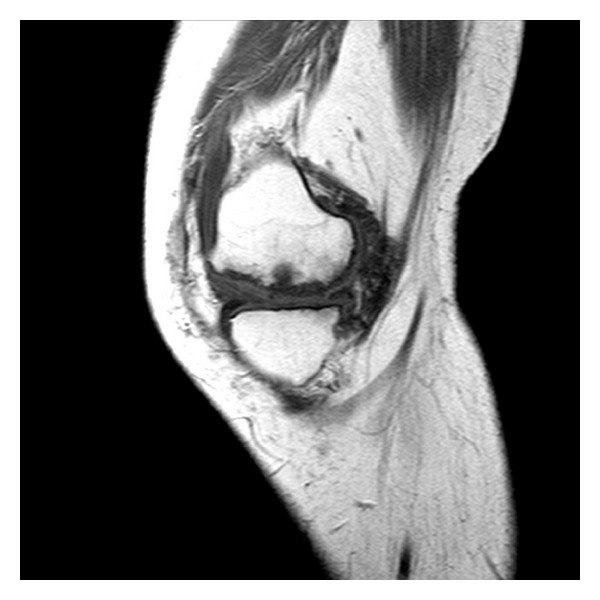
MRI T1 sagittal view of the left knee.
